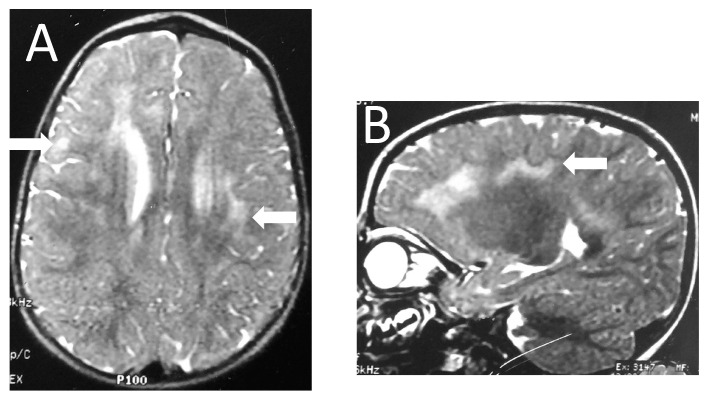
Patient 5. Axial (A) and sagittal (B) T2-weighted images show frontotemporal and parietal foci of high signal intensity (arrows) with patchy and asymmetric distribution.
